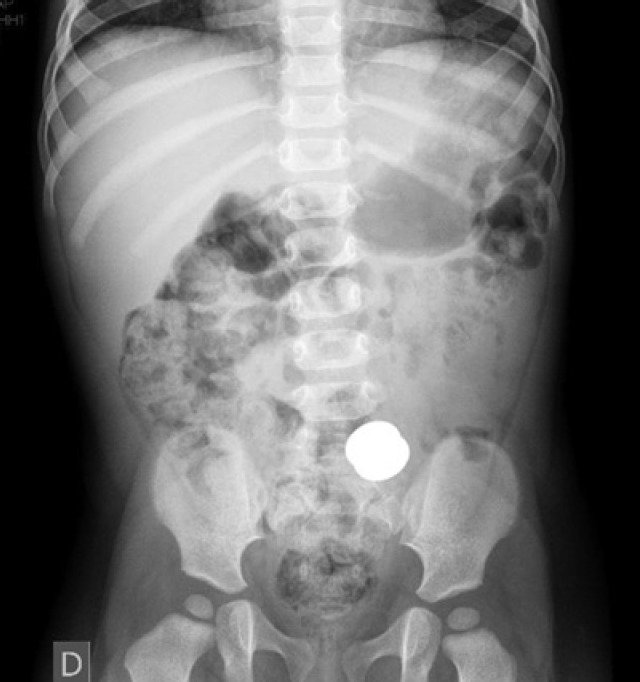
Simple abdominal radiograph showing a foreign body
